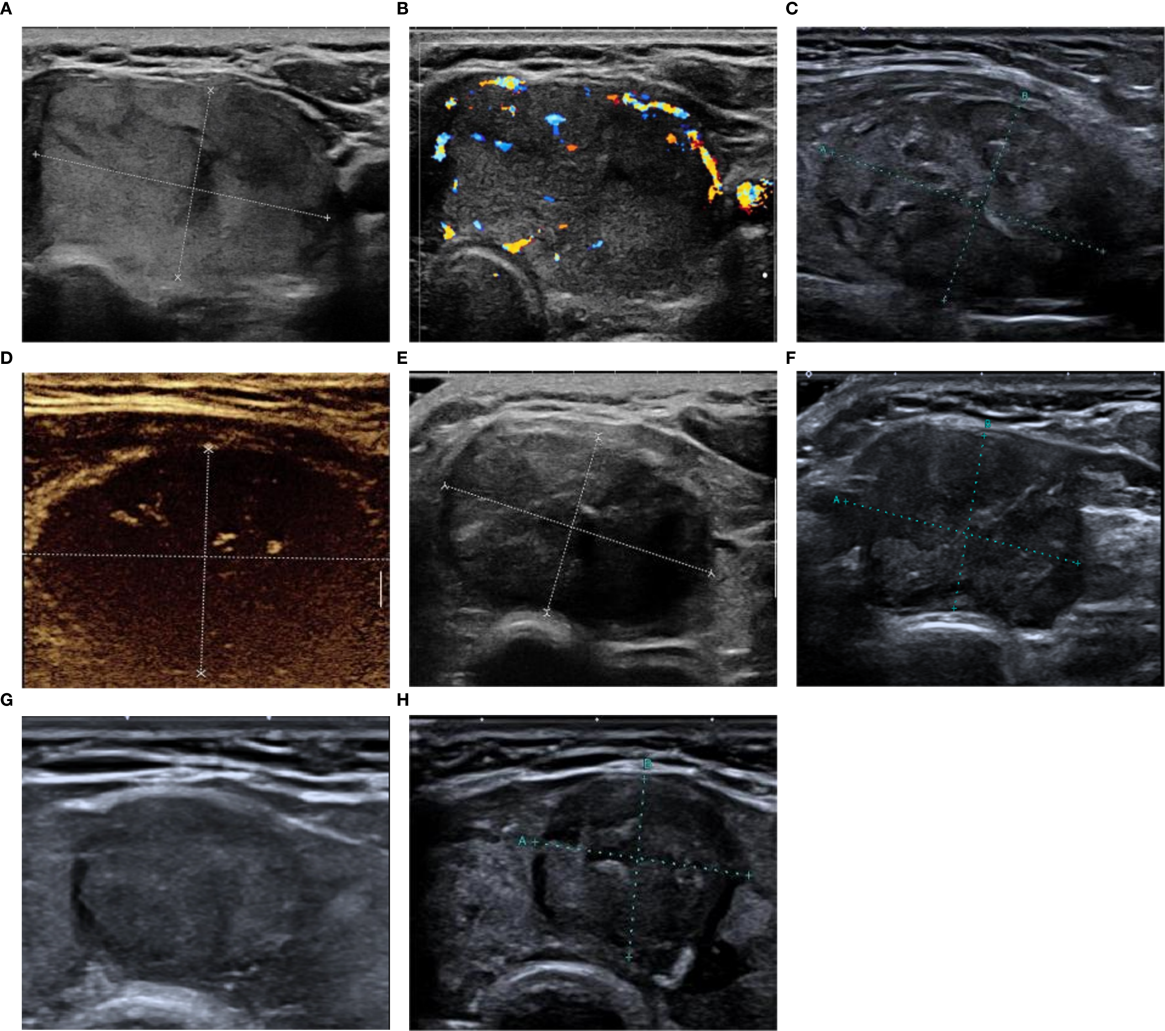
The patient is a 49-year-old female. (A, B) Preoperative ultrasound reveals large nodules in the thyroid isthmus and left lobe, which are isoechoic, with clear borders, Adler blood flow grade III, no calcification, size 3.92 cm × 2.05 cm × 4.04 cm, and volume 16.9 mL; (C) Two-dimensional ultrasound immediately after MWA reveals an oval-shaped hypoechoic state with clear boundaries; (D) Contrast-enhanced ultrasound examination immediately after MWA reveals no perfusion within the nodule; (E-H) Two-dimensional ultrasound images obtained at 1, 3, 6, and 12 months after treatment, respectively. The lesion volume seems to have decreased gradually, and VRR is 78.5% at 12 months after ablation. MWA, microwave ablation; VRR, volume reduction rate.
